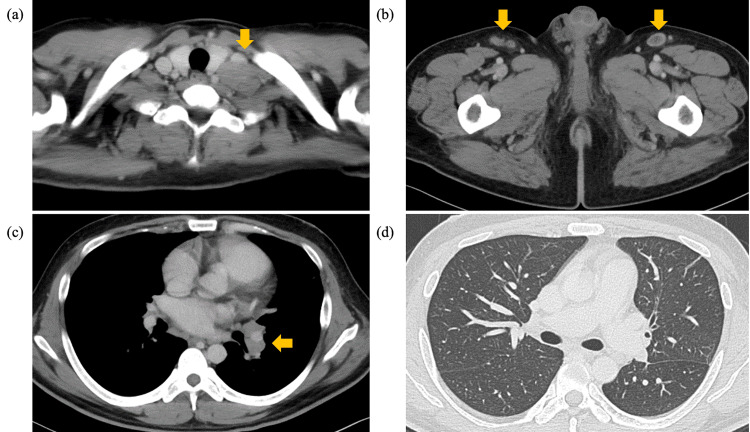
Computed tomography (CT) findings of the case on day 21. A contrast-enhanced CT scan was performed 21 days after the initial visit to our hospital. (a) Contrast-enhanced CT indicated an enlarged left supraclavicular lymph node, measuring 30 mm (yellow arrow). (b) Lymphadenopathy was observed in the bilateral inguinal regions (yellow arrows) and (c) in the left hilar region of the lung (yellow arrow). The left inguinal lymph node exhibited low attenuation internally, whereas the other lymph nodes were homogeneous, without contrast enhancement or low-attenuation areas suggestive of necrosis. Lymph nodes were visible in the axillary, mediastinal, and abdominal regions, but without definite pathological enlargement. (d) High-resolution chest CT revealed multiple small nodules randomly distributed throughout the lung fields, suggesting a hematogenous lesion.
